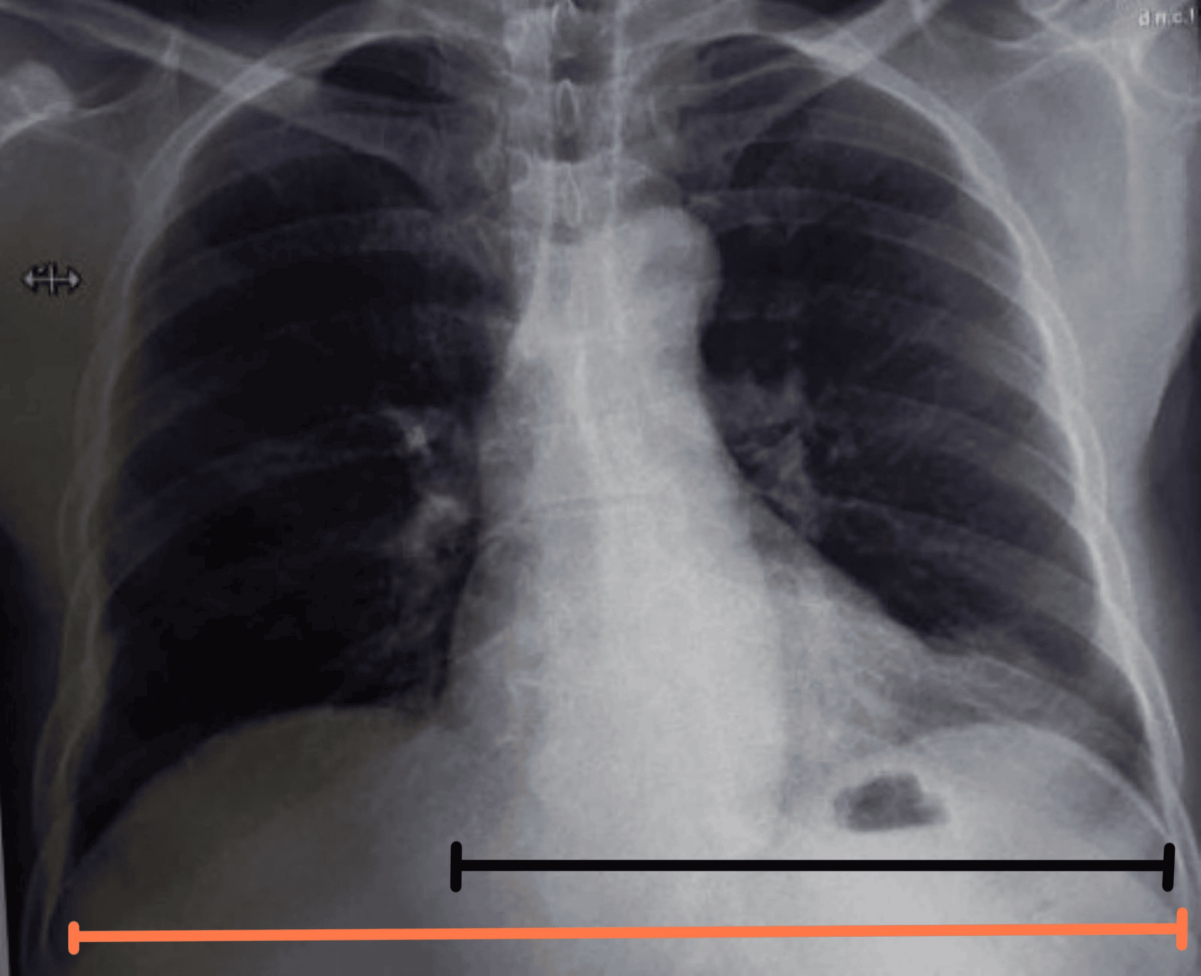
Chest X-ray posteroanterior view showing increased cardiothoracic ratio suggestive of cardiomegaly The cardiothoracic ratio is the ratio of the cardiac margin (black line) divided by the thoracic margin (orange line). A ratio of more than 0.5 in adults suggests cardiomegaly
